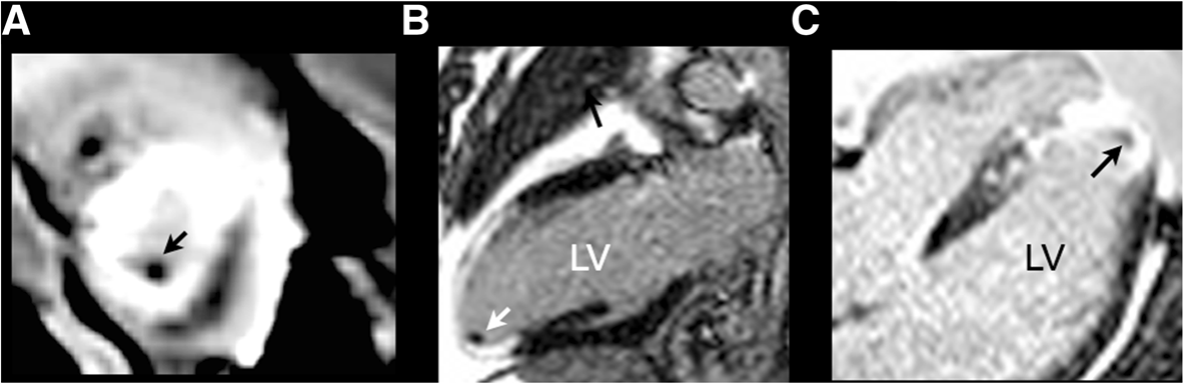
Delayed enhancement cardiac magnetic resonance imaging sequence in the short axis view (A), apical 2 chamber view (B), and apical 4 chamber view (C) confirming full thickness scar involving the mid to distal anterior wall and apical regions, with delineation of a small left ventricular apical thrombus measuring 10 x 5 mm in dimension.
